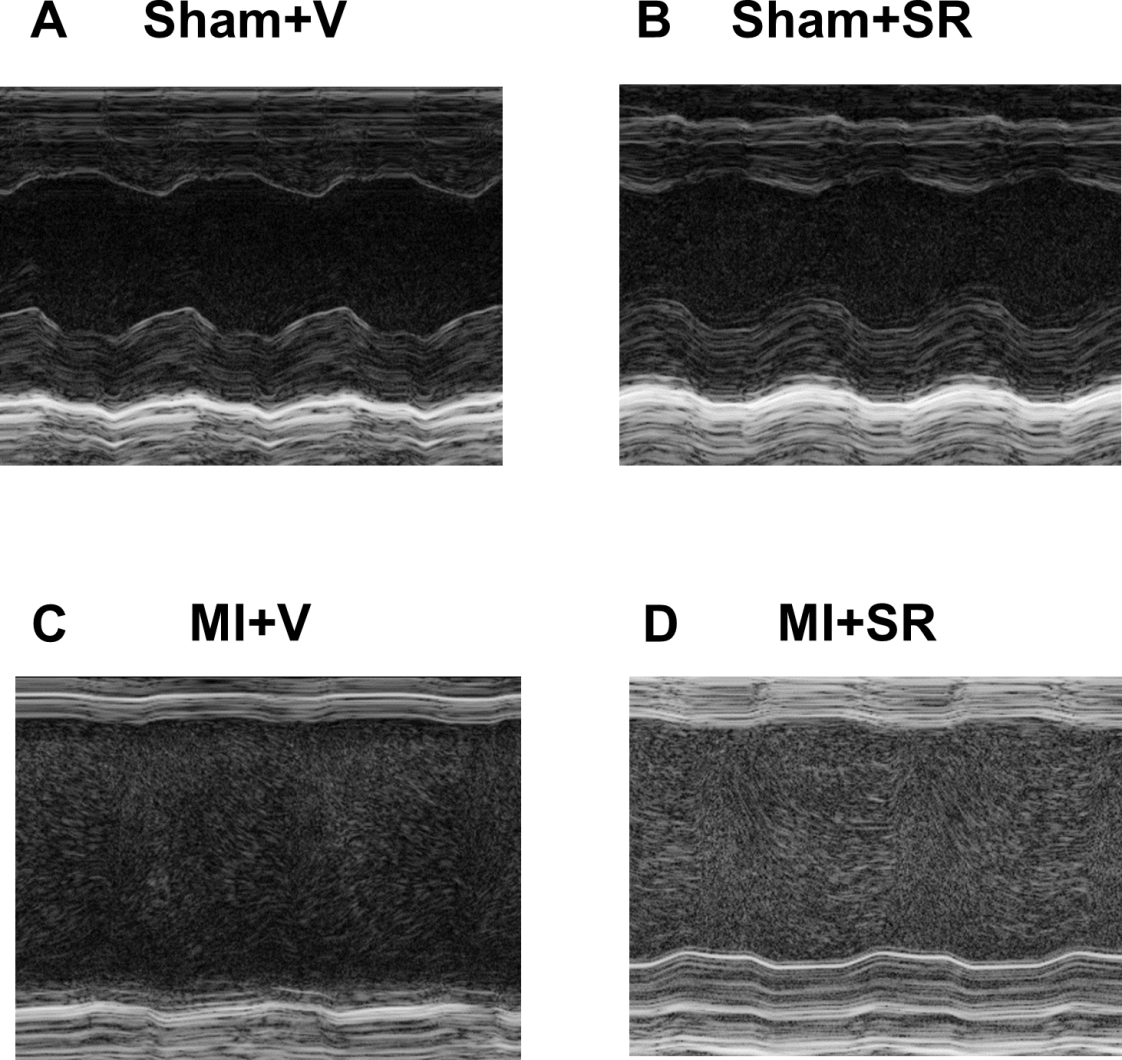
M-mode echocardiographic analysis. The representative M-mode findings of the left ventricular short-axis view on day7 after MI: (A) Sham+V, (B) Sham+SR, (C) MI+V, and (D) MI+SR.
